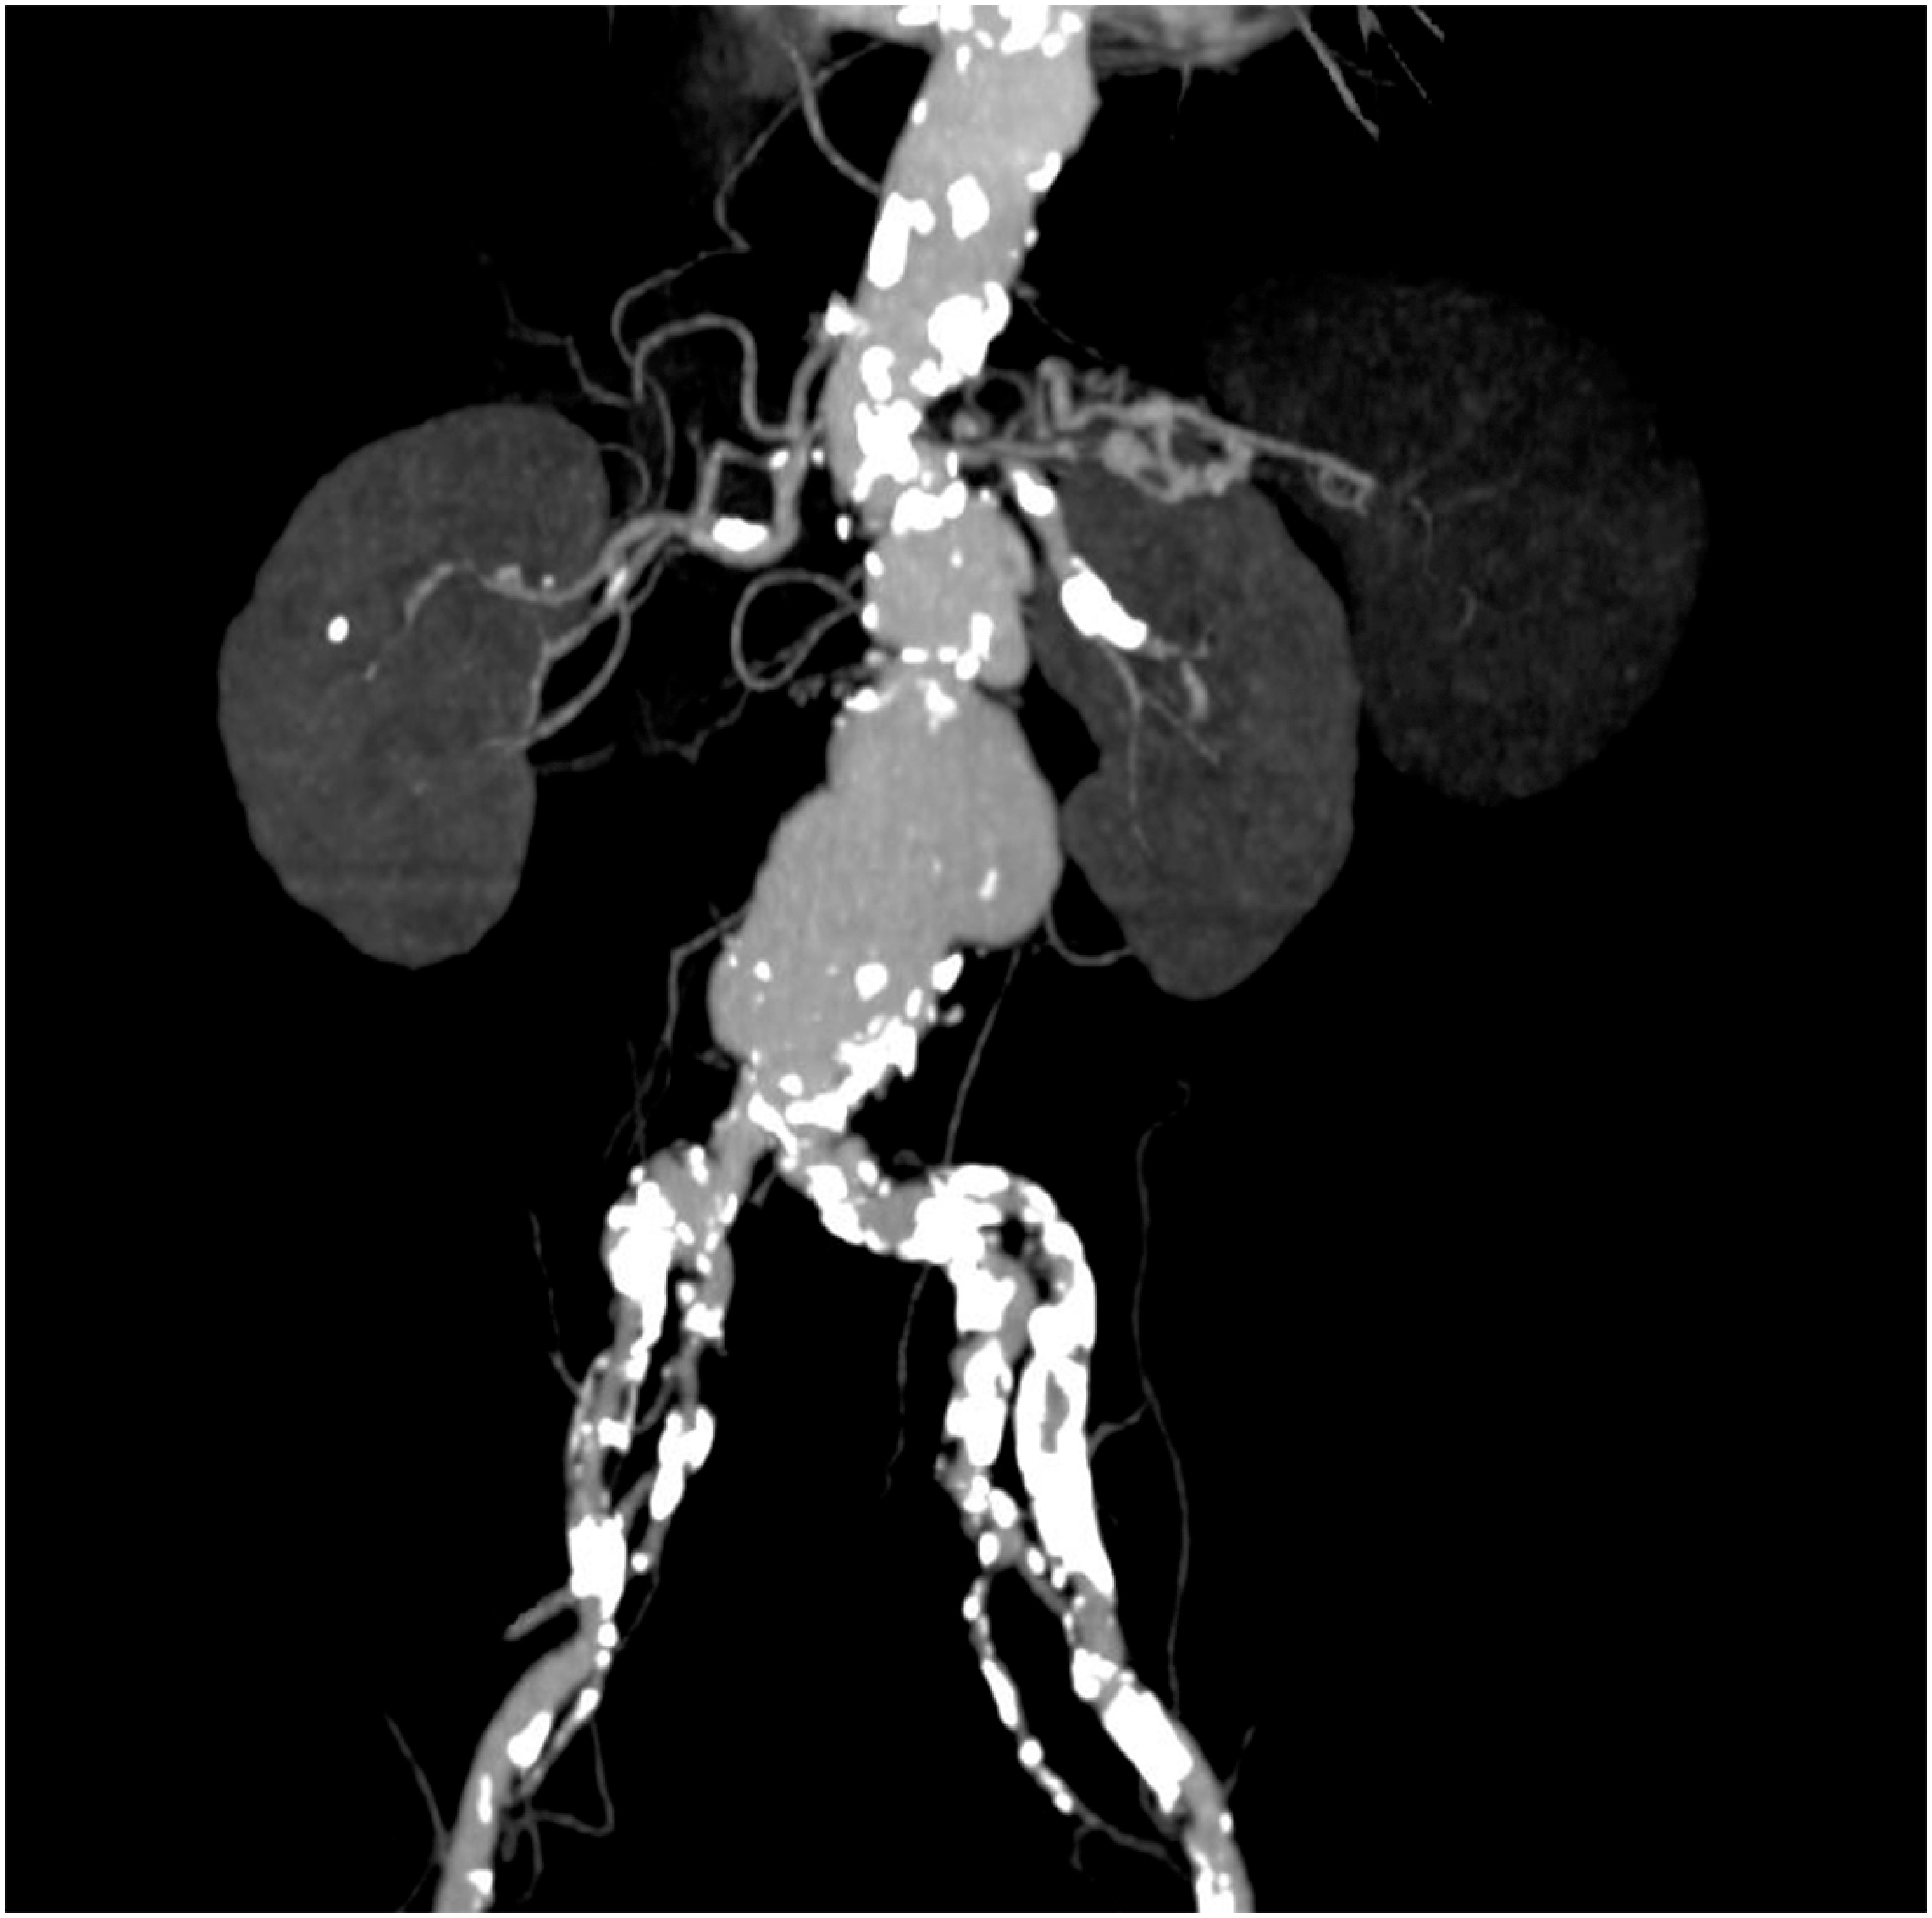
Fig. 1 Contrast-enhanced computed tomography of the aneurysm.
